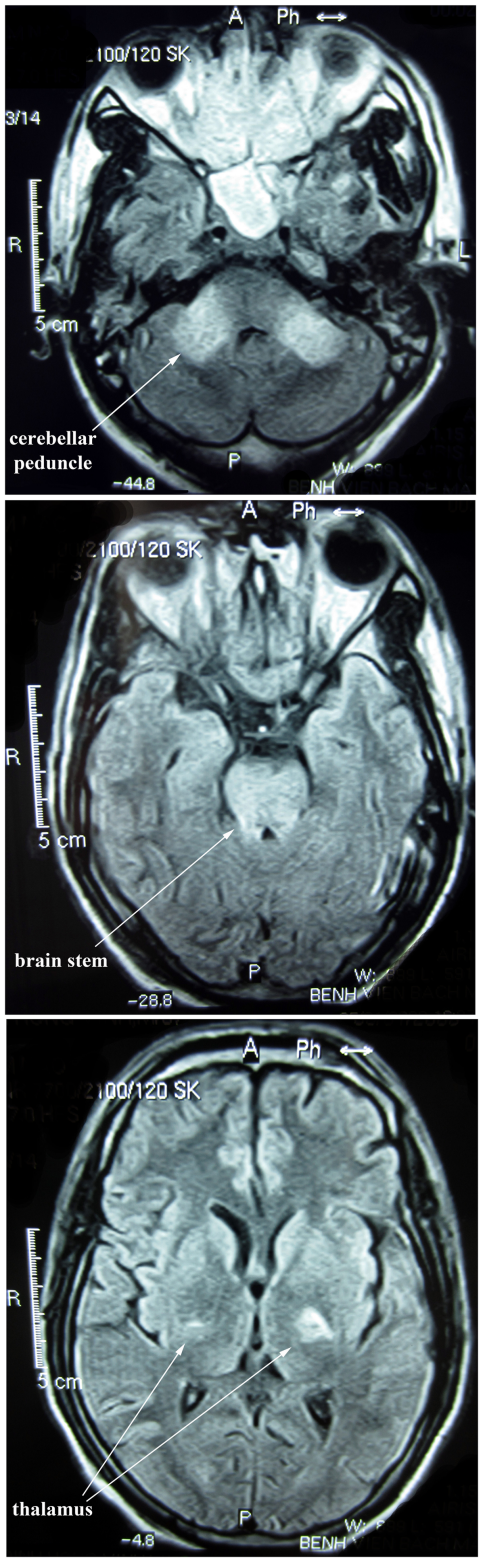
Brain MRI images for an AME patient scanned 9 days after the onset of confusion. FLAIR images show high-intensity signals in the cerebellar peduncle, brain stem and thalamus.
